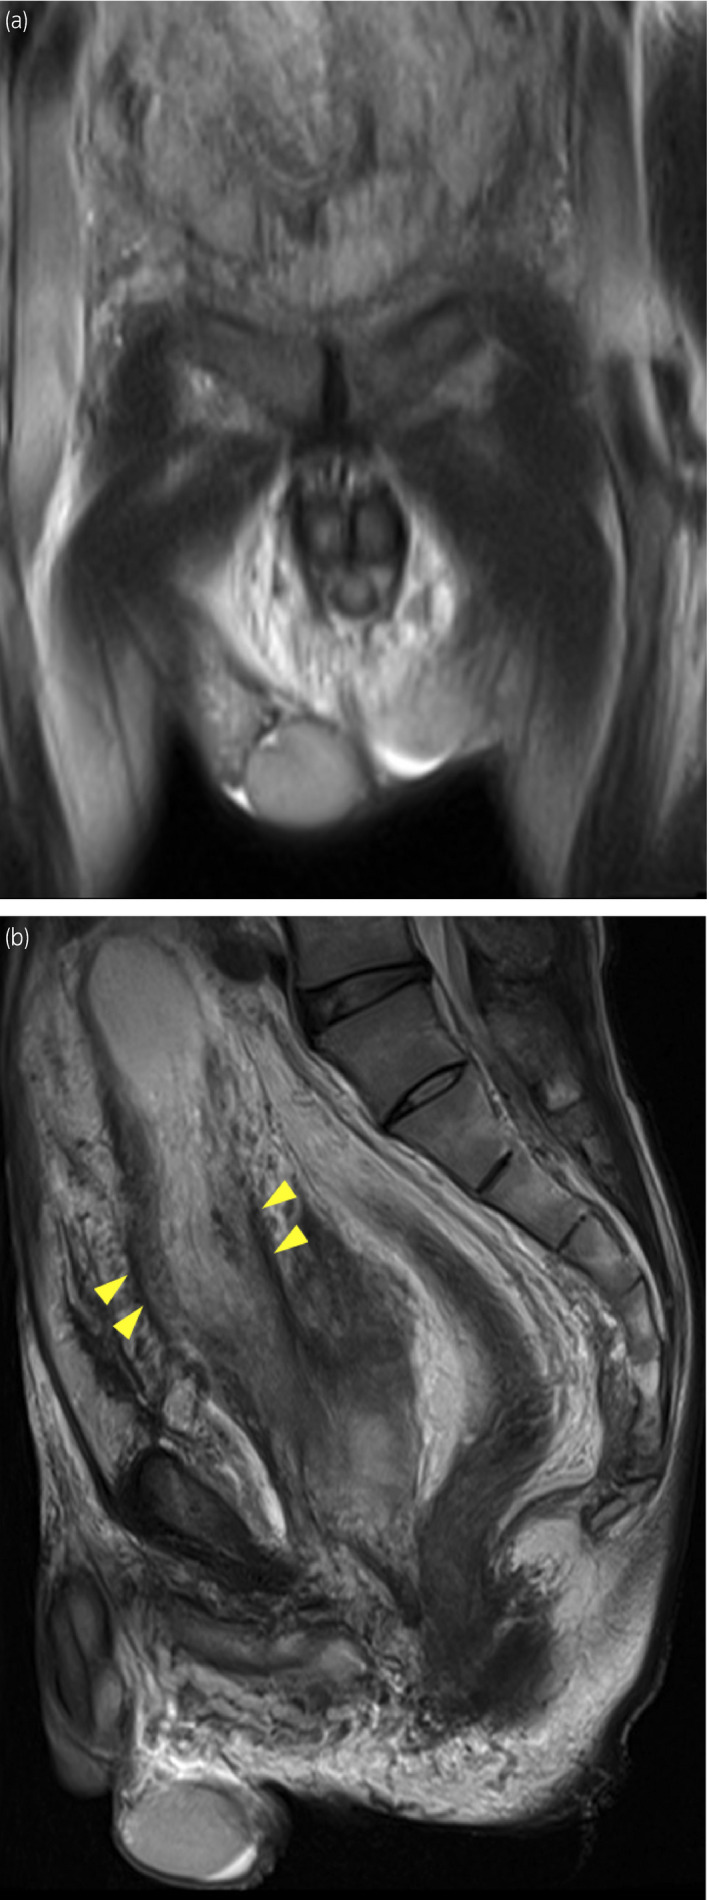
(a) MRI a year ago showing normal right testis. (b) MRI a year ago showing a thickening and poor dilation of the bladder wall from the neck to the trigone. The thickened bladder wall is indicated by arrowhead. The size of the right testis is 3.3 × 2.4 cm.
